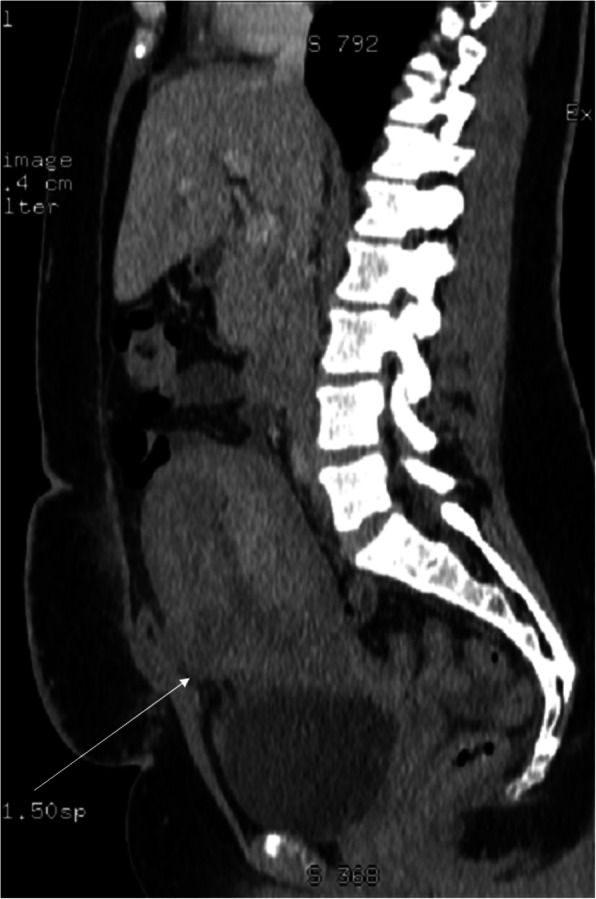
A 34-year-old primigravida primipara 12 days after C-section complicated by dissection of the posterior wall of the uterus. CT in a venous phase shows the normal postoperative appearance of a recent cesarean delivery incision which is hypodense as compared to the intact myometrium (thin arrow)
